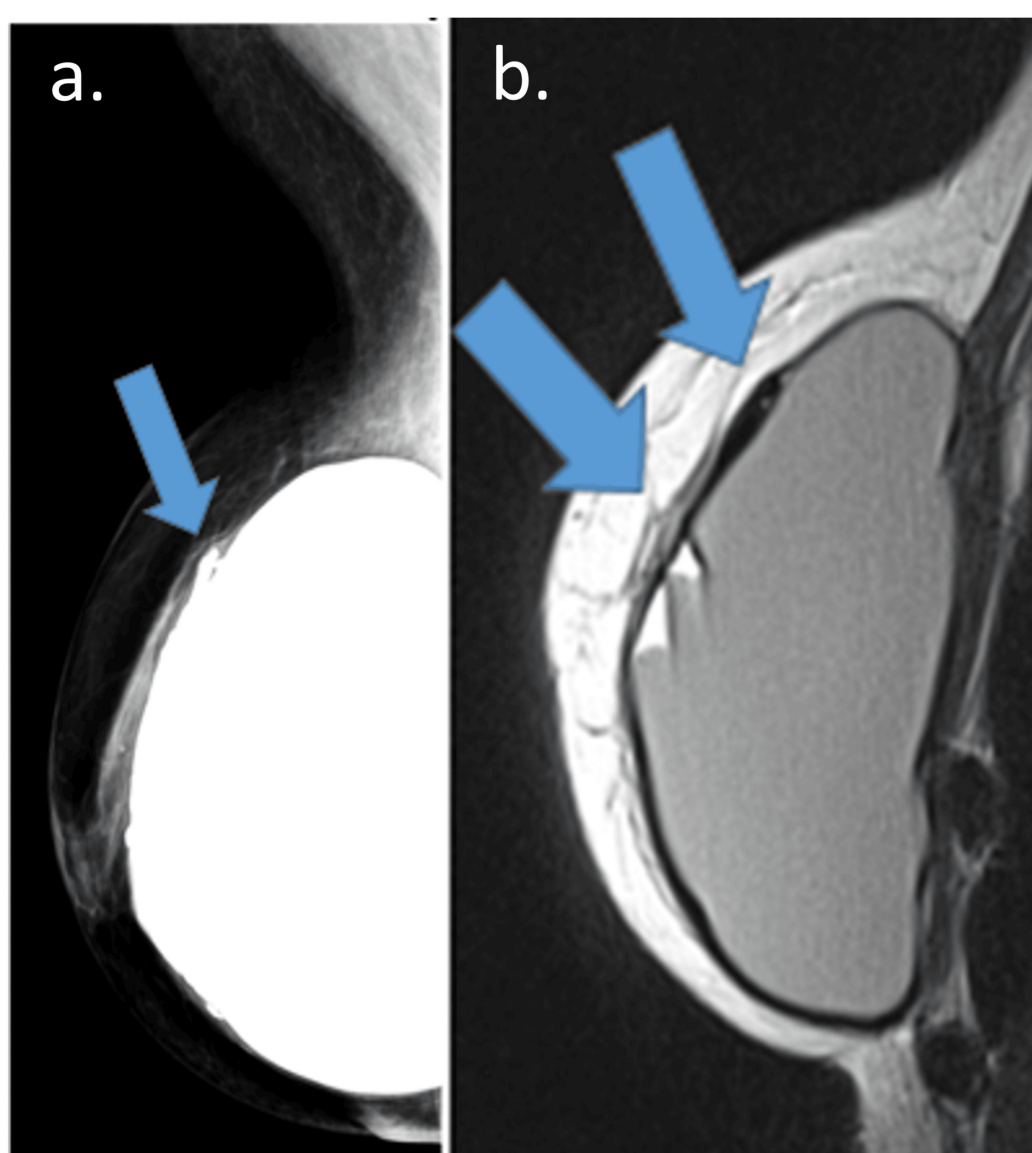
Late complication of acelullar dermal matrix A 42-year-old woman with an adenomastectomy for 10 years. (a) Mammography oblique view and (b) sagittal PD sequence image (a and b) Gross calcification in the pericapsular space (blue arrow) on mammography and MRI
